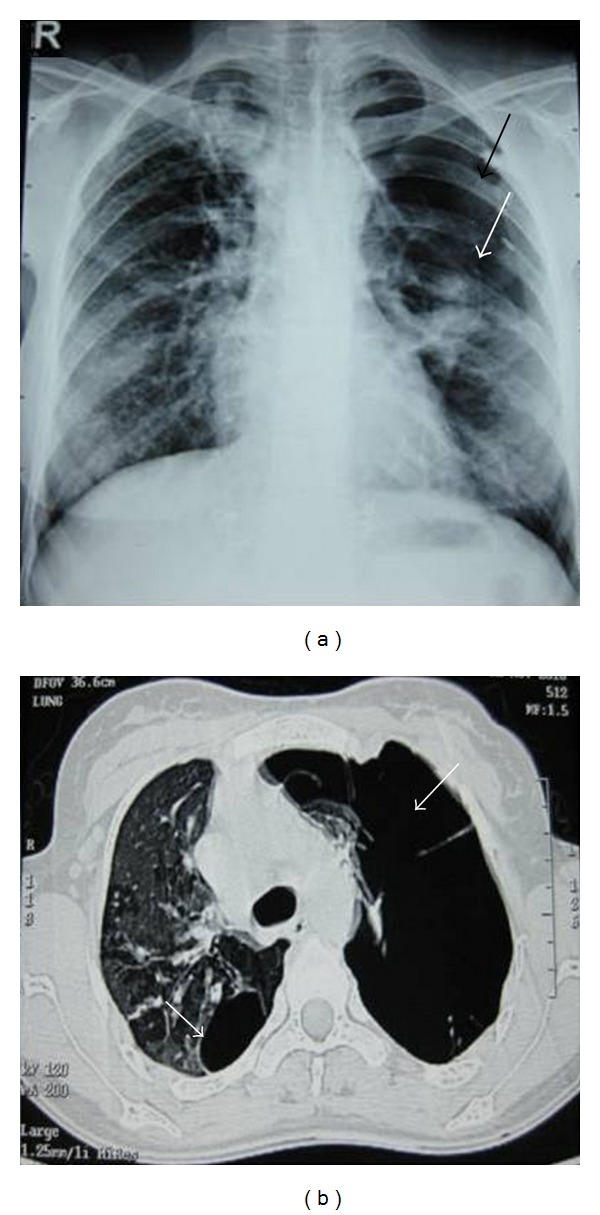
Chest X-ray (a) in the Emergency Department showing pneumothorax of left side (black arrow) with collapsed visceral pleural line (white arrow) and corresponding computed tomography of thorax (b) showing massive pneumothorax of left side (white arrow) with infiltrates and cystic space (white arrow) in right upper lobe.
